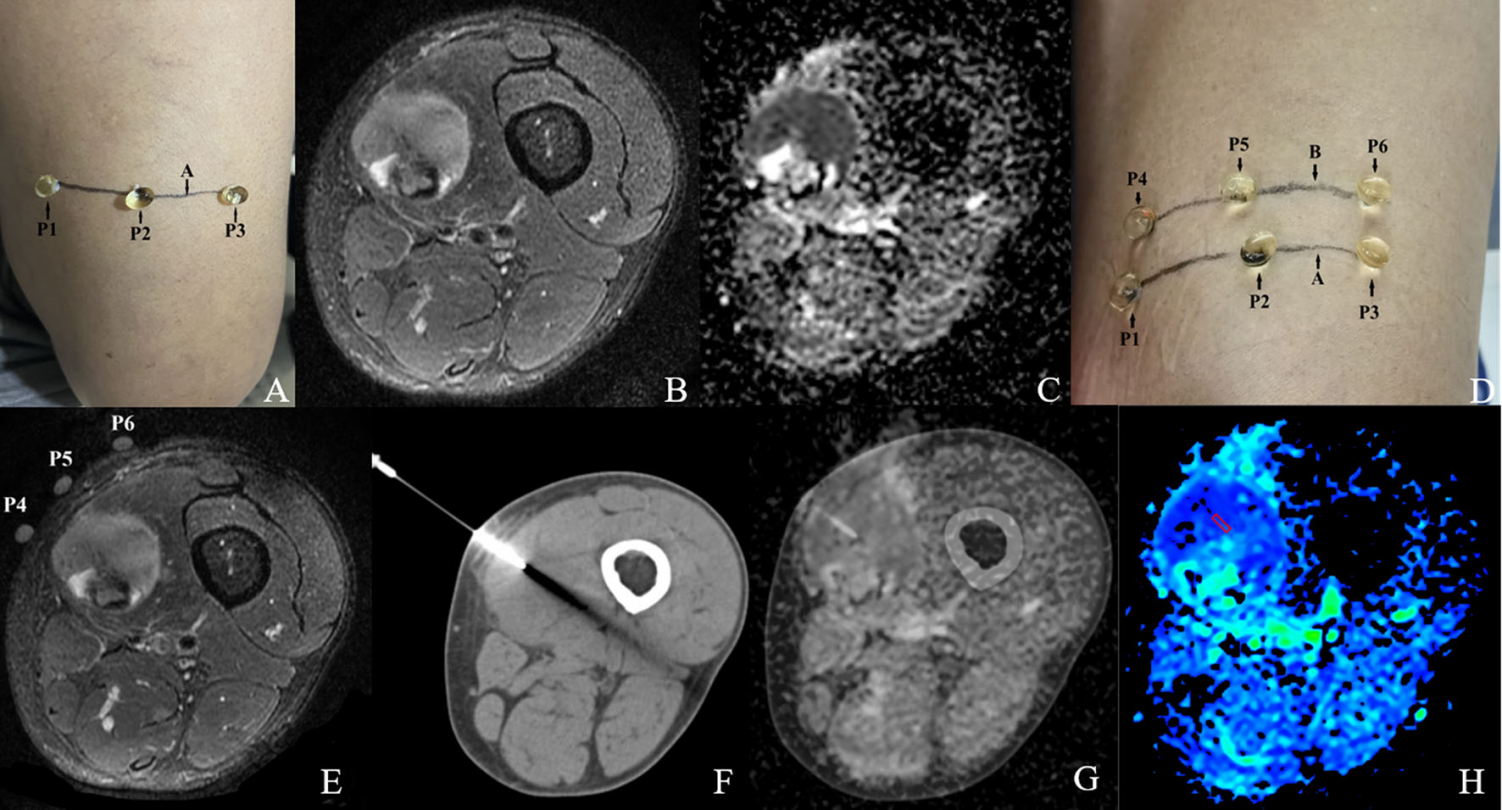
CT-MRI image fusion registration method.
